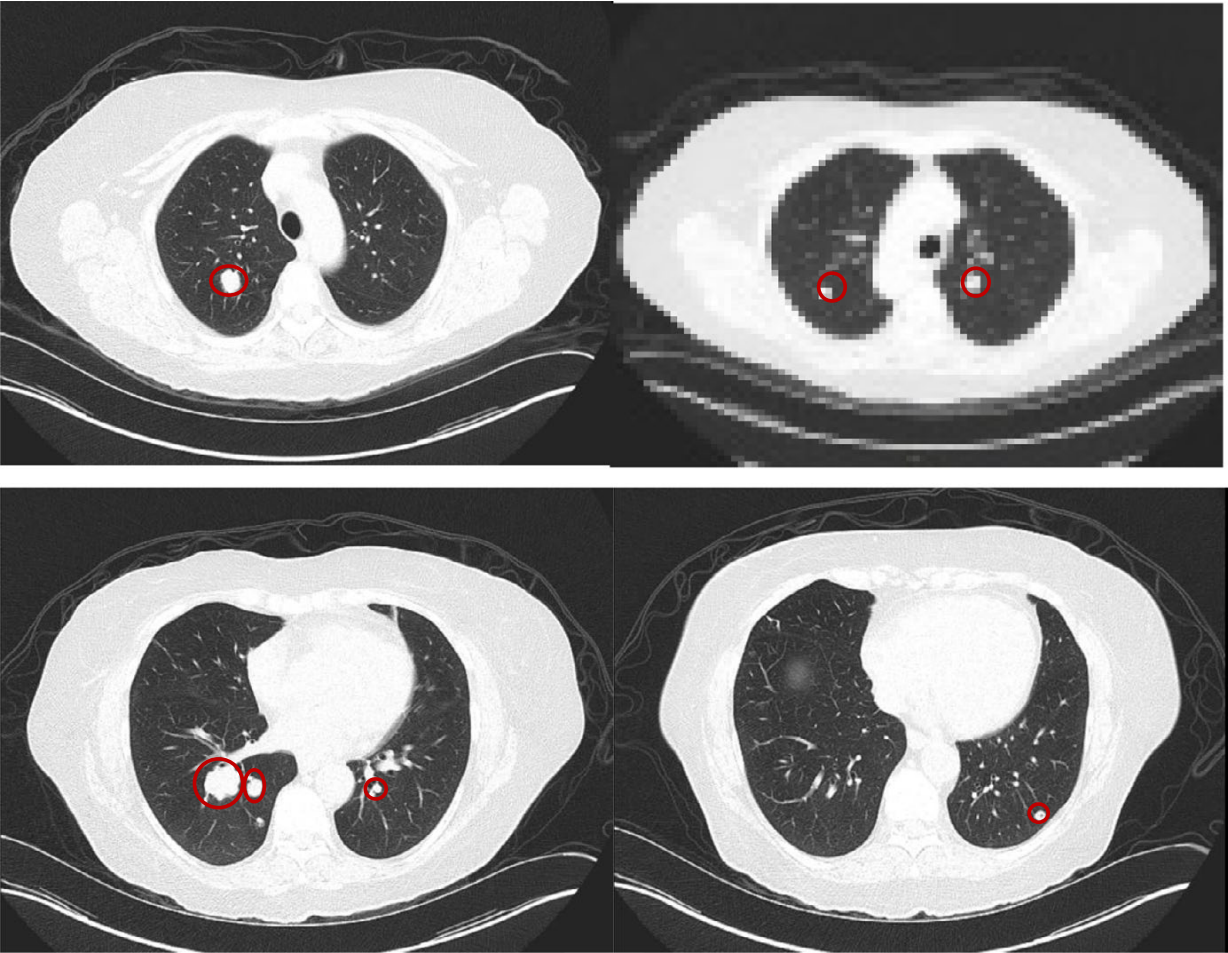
CT scans of the patient before modified‐ docetaxel, cisplatin, and fluorouracil (DCF) chemotherapy showing multiple lung metastases
